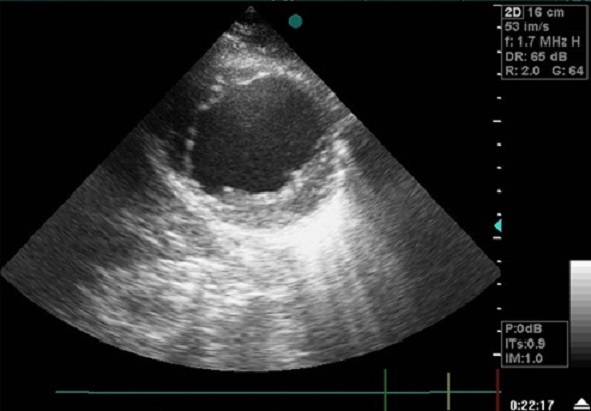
Coupe parasternal petit axe à l'ETT montrant un volumineux anévrysme du VG
